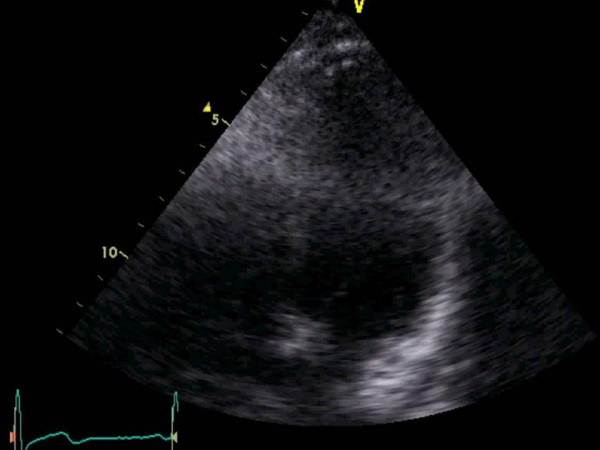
Similar to figure 2, a large shadow is seen across the right and left ventricle secondary to the silicone breast implant in this patient limiting echocardiographic window in the 4 chamber view.
